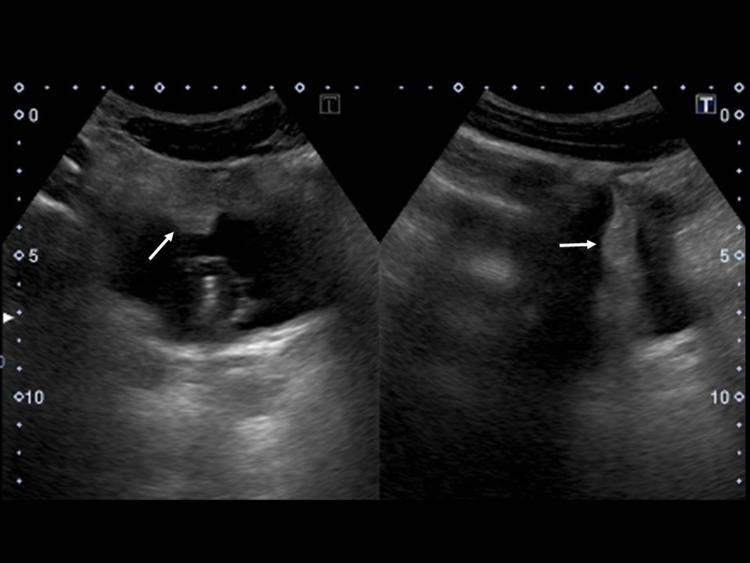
Ultrasound findings on day 2 Ultrasound on day 2 also indicated trabecular formation (arrow) in the bladder.
